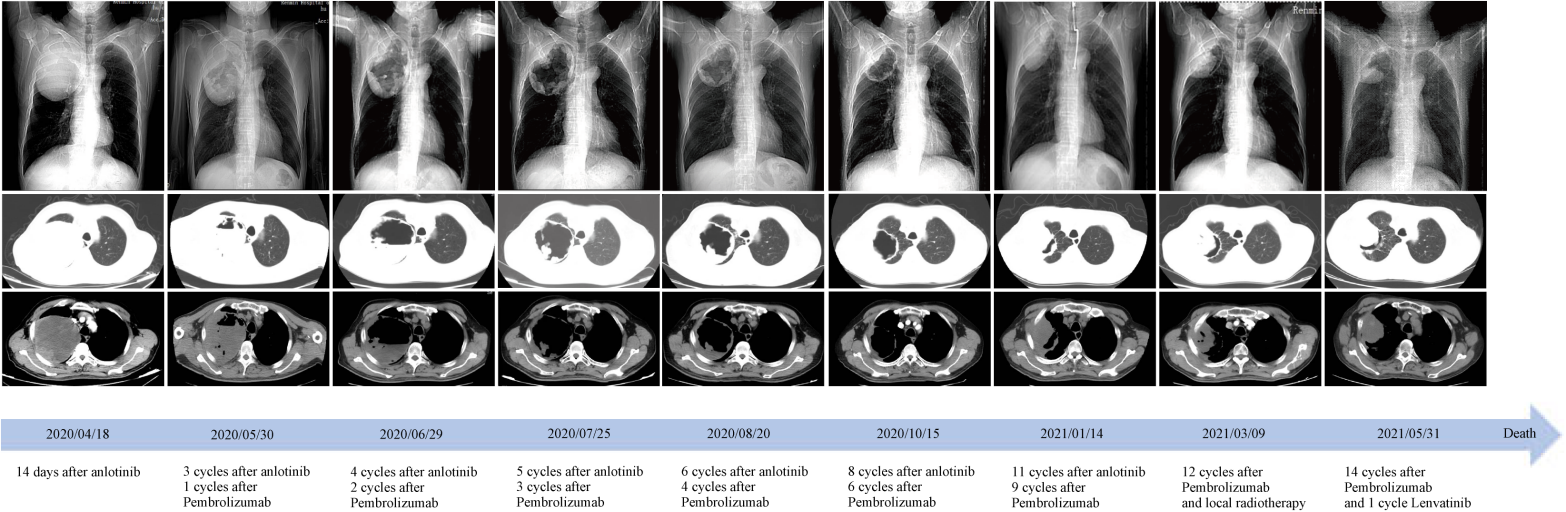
Time course of treatment and changes in the lung CT scans during treatments. Coronal and axial CT scans showed that the central necrotic area of the right upper lobe mass gradually became larger and more cavernous, and the solid cavity wall gradually became thinner. After 9 months of treatment, the right upper lobe mass began to enlarge and invade the chest wall and axilla. CT, computed tomography.
